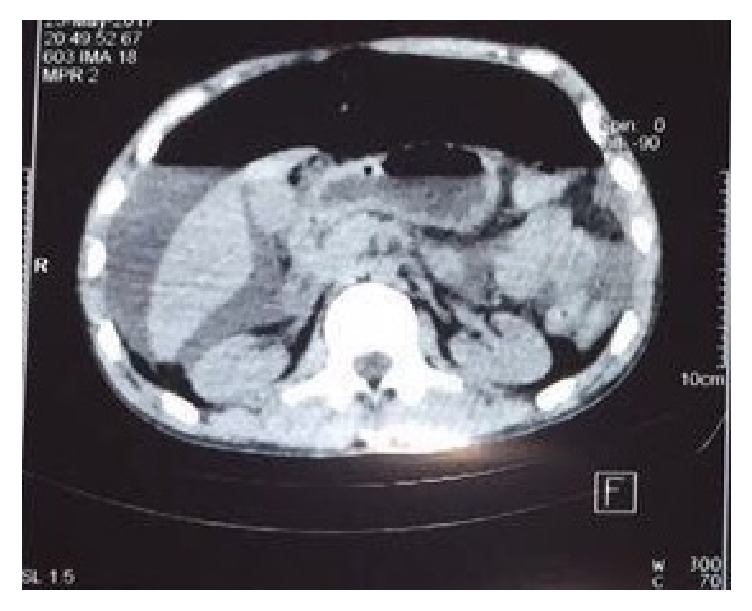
CT abdomen image demonstrating free fluid to the right upper quadrant and free air.
